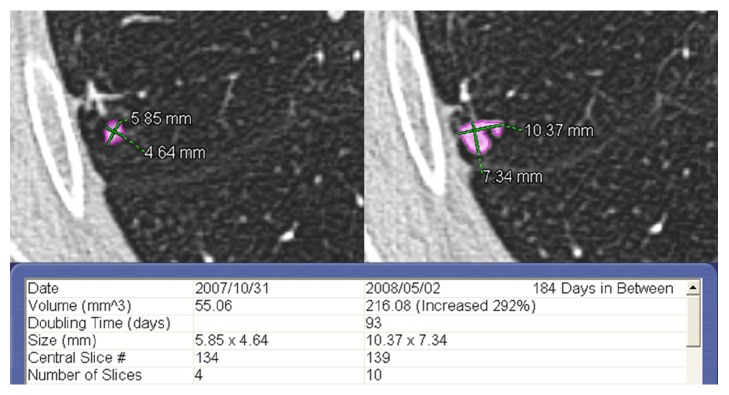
Solid pulmonary nodule smaller than 100 mm^3^ with smooth margins located in the right upper lobe in a 71-year-old high-risk male patient. Baseline (left) and follow-up CT scans (right). The interval between the two CT examinations was 184 days. The software calculated significant growth between the baseline and follow-up CT scans with a relative volume variation of 292% (from 55 mm^3^ to 216 mm^3^) and a volume doubling time of 93 days. The nodule was surgically removed and proven to be a pulmonary adenocarcinoma. In this case, the new Fleischner Society guidelines would have recommended a follow-up CT examination at 12 months.
